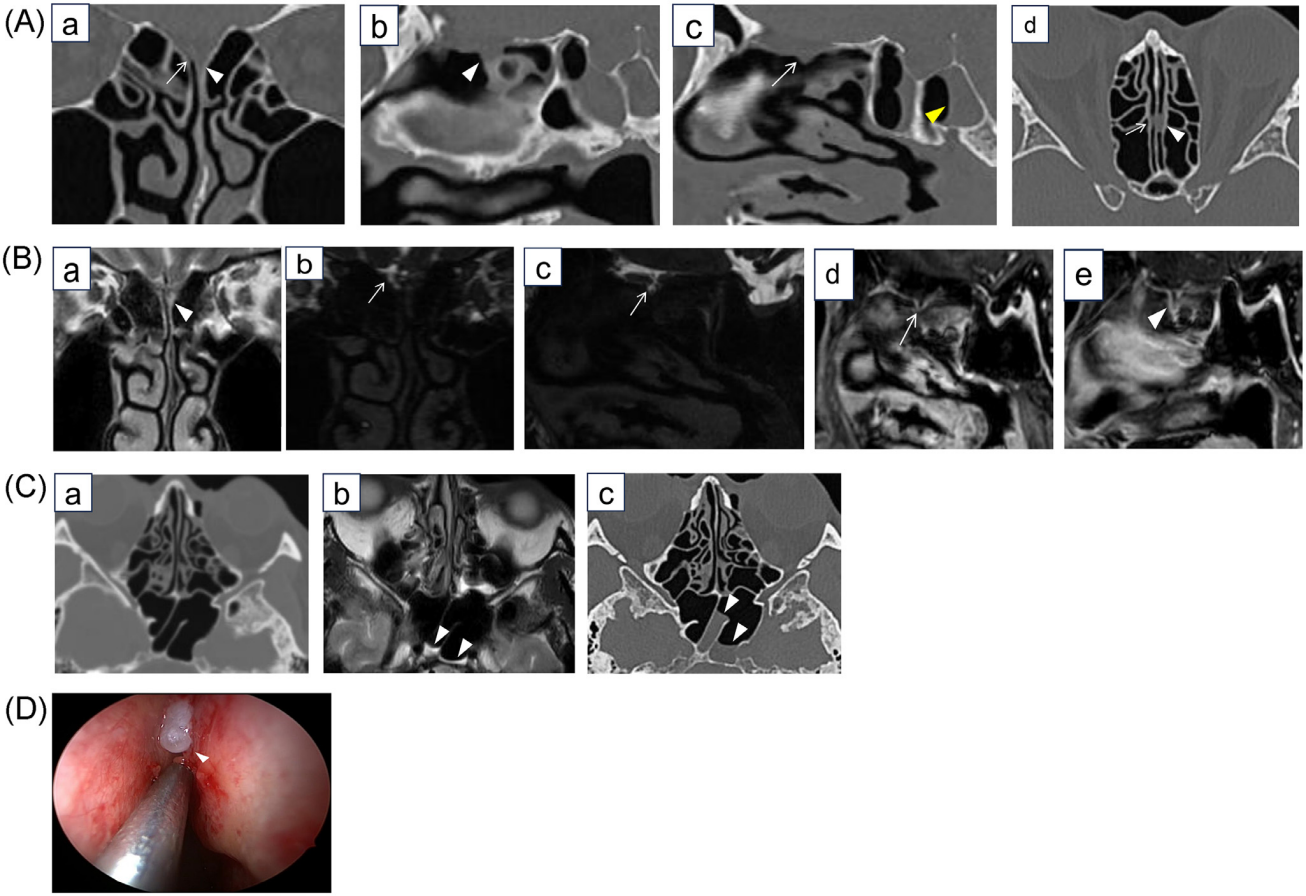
CT (a) Oblique coronal plane aligned perpendicular to the base of the skull. (b) Oblique sagittal plane aligned with the left olfactory fissure. (c) Oblique sagittal plane aligned with the right olfactory fissure. (d) Oblique axial plane aligned parallel to the base of the skull. Bilateral cribriform plates were indistinct, but it was unclear whether there were bone defects (a-c). A soft tissue density protruded from the cribriform plate in both olfactory fissures with left-sided predominance, which may have indicated the site of leakage (a-d). CSF leakage from the left side was suspected on the basis of the patient's symptoms and an air-fluid level in the left sphenoid sinus (c) (yellow arrowhead). 1B. MRI. (a) Coronal T2WI. (b) Coronal 3D-MR cisternography. (c) 3D-MR cisternography oblique sagittal plane aligned with the right olfactory fissure. (d) 3D-MR cisternography oblique sagittal plane aligned with the right olfactory fissure. (e) Gd-T1WI oblique sagittal plane aligned with the left olfactory fissure. Coronal T2WI showed a high signal intensity in the left olfactory fissure, which corresponded to the soft tissue density seen on CT (a) (arrowhead). On 3D-MR cisternography, the signal in the left olfactory fissure was unclear, and there was a high signal intensity continuous from the subarachnoid space to the right olfactory fissure (b and c) (arrows). Gd-TIWI showed a meningocele-like structure protruding from the cribriform plate on both sides (d and e) (arrow and arrowhead). 1C. (a) Axial CT image. (b) Axial T2WI MRI 3 hours after the CT images were obtained. (c) Axial CT image 4 days after the MRI images were obtained. (d) The air-fluid level in the left sphenoid sinus increased in size between the CT and MRI imaging, suggesting persistent leakage of CSF in the left cribriform plate (a-c) (arrowheads in b and c indicate the accumulating fluid collection). No air-fluid level was observed in the right sinus. 1D. Endoscopy A meningocele (arrowhead) measuring 1-2 mm in size was found in the left olfactory fissure during endoscopic surgery. CT, computed tomography; CSF, cerebrospinal fluid; MRI, magnetic resonance imaging; T2WI, T2-weighted imaging.
